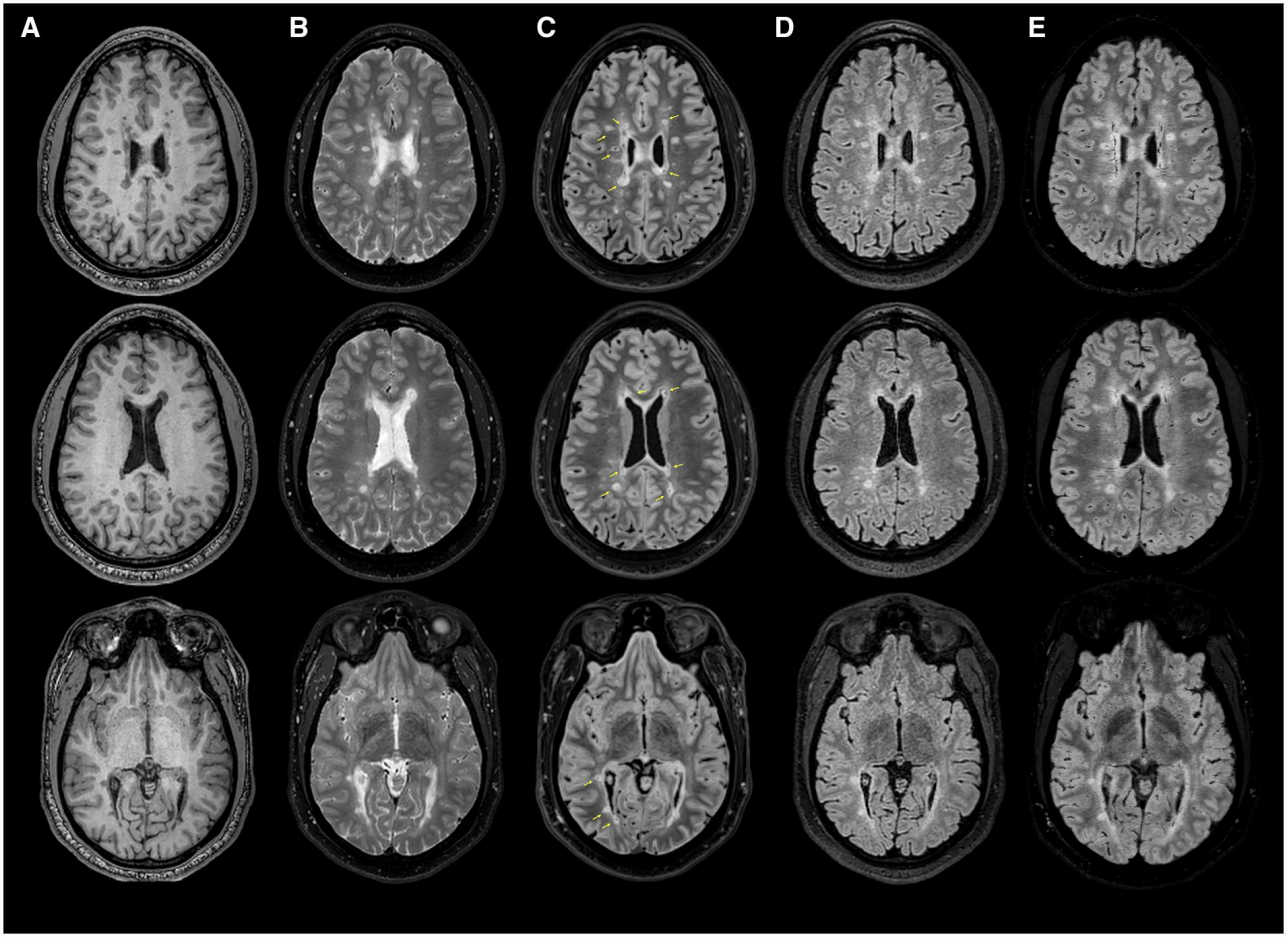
Select sequences from a 3-Tesla MRI of the brain. (A) Axial T1-weighted and (B) T2-weighted images of the brain revealing multi-focal regions of signal abnormality in locations typical for multiple sclerosis (MS). (C) Axial T2-fluid attenuated inversion recovery (FLAIR) fat-suppressed images demonstrating regions of T1-hypointensity within lesions (yellow arrows). Note the different characteristics in lesions from the FLAIR imaging sequence (D) and the fat-suppressed T2-FLAIR mismatch (B compared to C). (E) Axial FLAIR star MRI images of the brain highlighting the “central vein sign” within selected lesions in (C). The number of lesions with central vessels present suggest an origin of MS.
